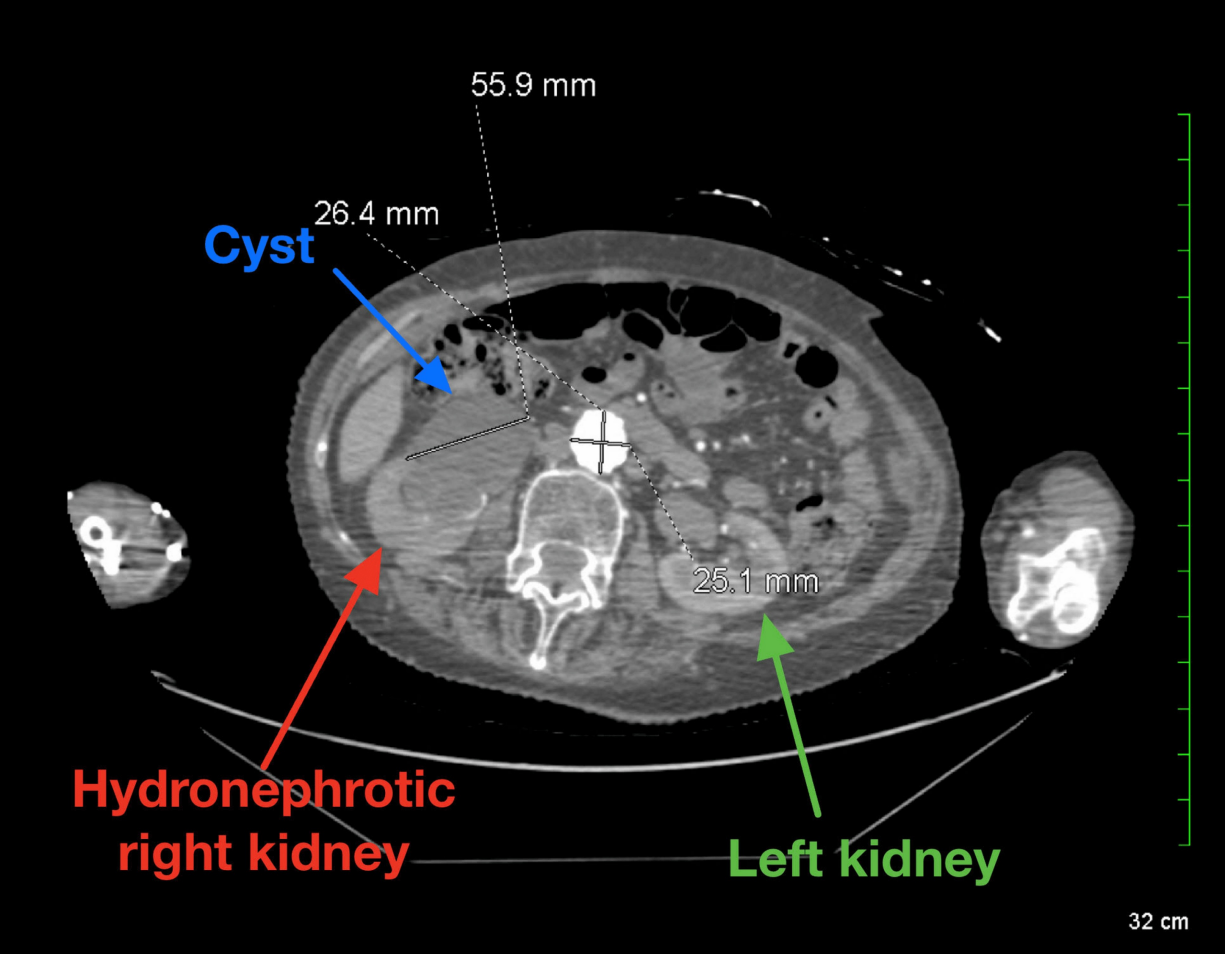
CT angiography of the abdominal region demonstrating right kidney hydronephrosis (red arrow) and a cyst (blue arrow). CT: Computed tomography
